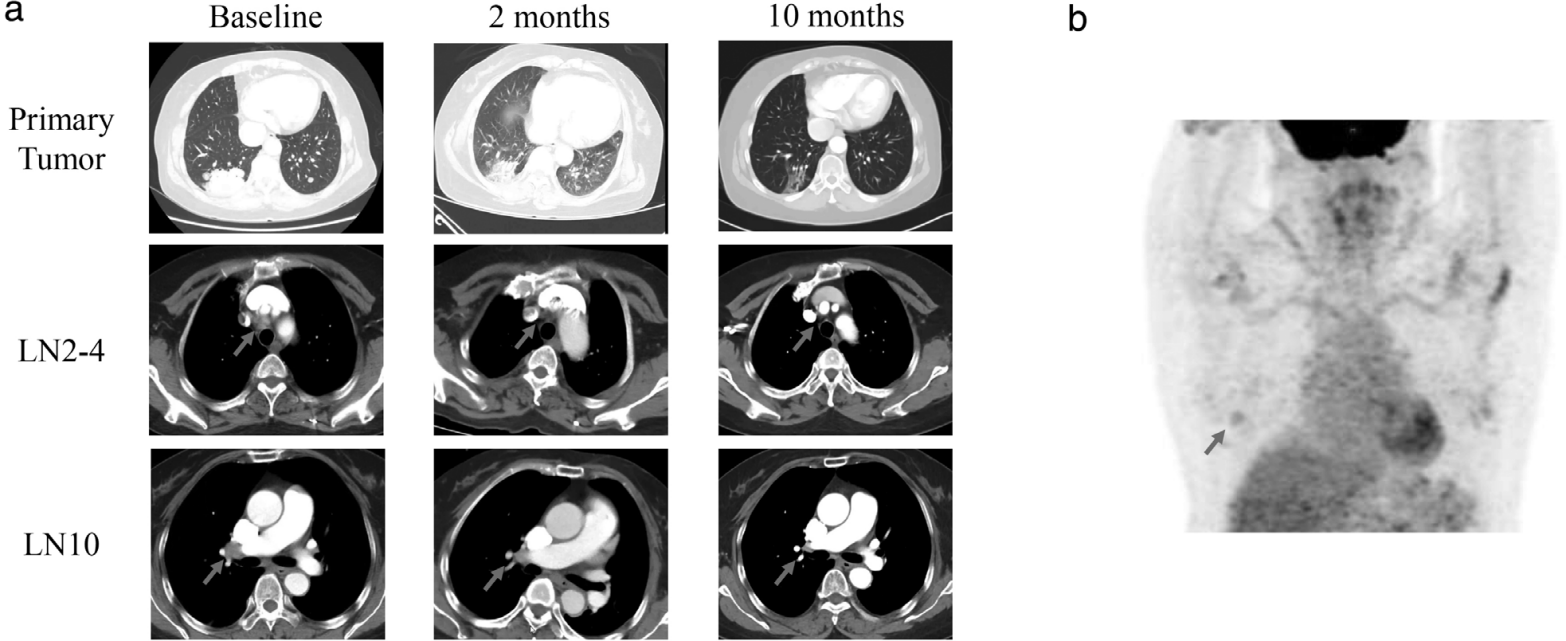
Images during neoadjuvant crizotinib treatment. (a) Enhanced chest CT images of right lung adenocarcinoma as well as lymph nodes (LN) prior, 2 and 8 months post crizotinib treatment; (b) PET‐CT image before the surgery
